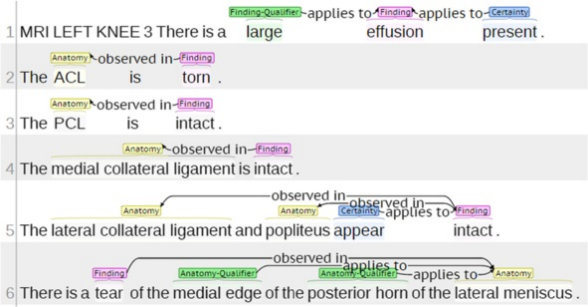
A manually annotated MRI report. A screenshot of the BRAT interface
